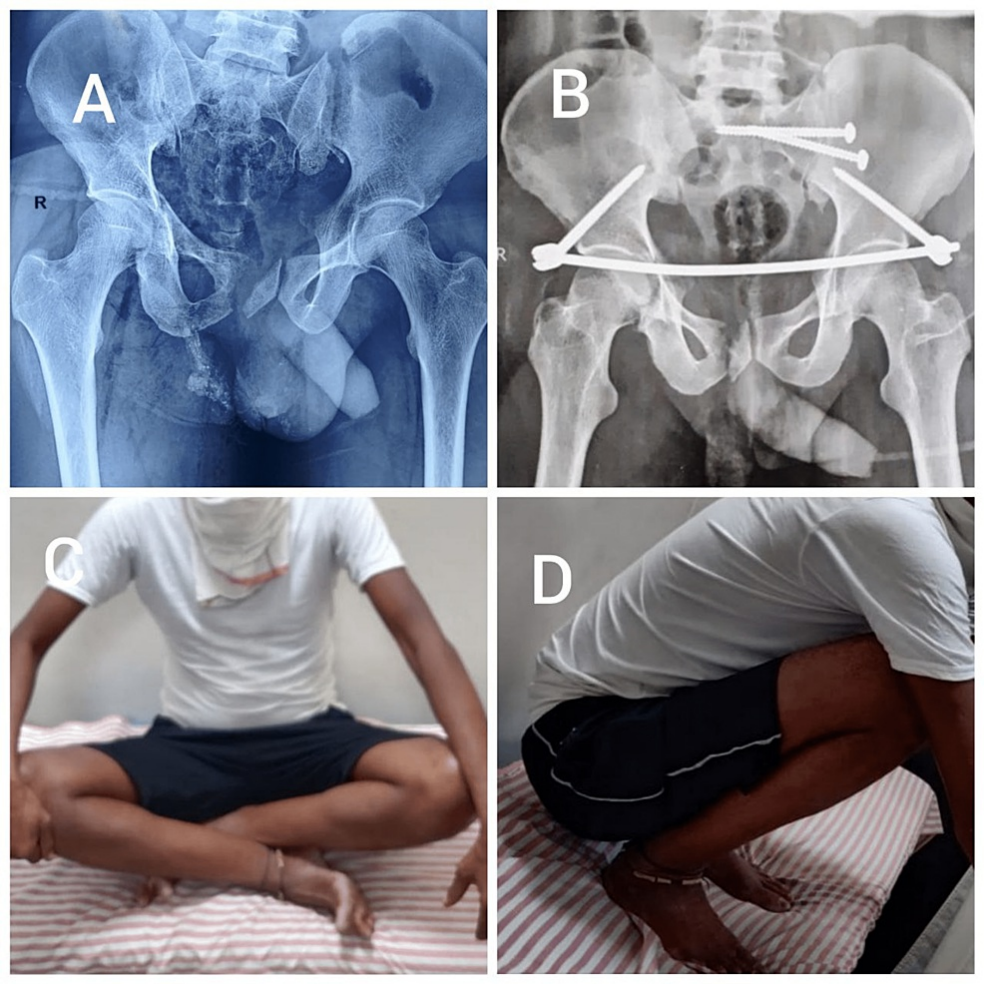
A) A 54-year-old male with anterior and posterior pelvic ring injury. B) Immediate postoperative X-ray. C and D) Range of motion at six months.
